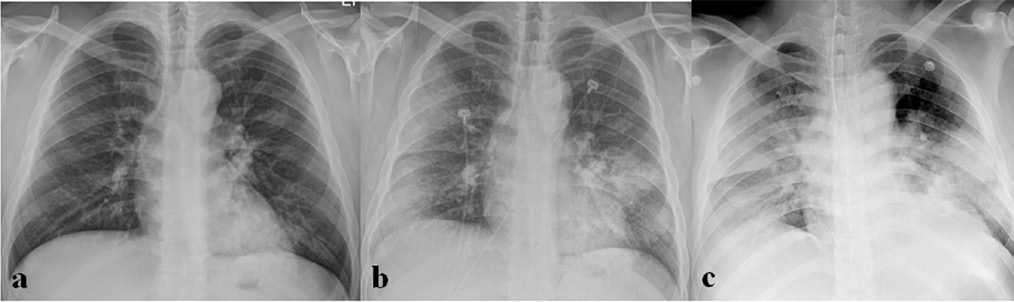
A 36-year-old male patient with MERS-CoV infection. He had a contact history of MERS patients and suffered from fever. (a) Initial chest radiograph (CXR) shows multifocal patchy consolidation in left middle to lower lung zones. (b) Follow-up CXR obtained 15 days after (a) shows increased extents of multifocal patchy consolidation in left middle to lower lung zones and new appearance of diffuse ground-glass opacities and consolidation in the peripheral portion of right lung. (c) Follow-up CXR obtained 18 days after (a) shows bilateral diffuse consolidation, suggesting acute respiratory distress syndrome.
